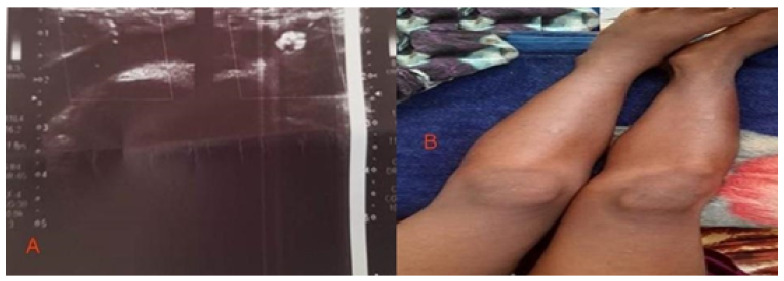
(A) Patient on initial presentation, Doppler ultrasound, and (B) after one week from management.
